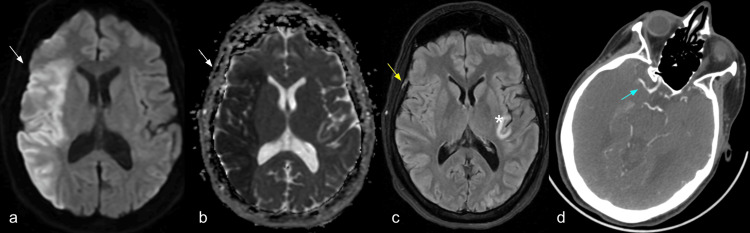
Brain magnetic resonance imaging study Images showing changes that indicate restricted diffusion in the vascular territory of the right middle cerebral artery (white arrows), without any representation on the T2/ FLAIR images (yellow arrow), findings in keeping with acute infarction. Additionally, the left insular region showed changes compatible with subacute infarction (asterisk) and occlusion of the distal portion of the M1 segment of the right middle cerebral artery (blue arrow).
